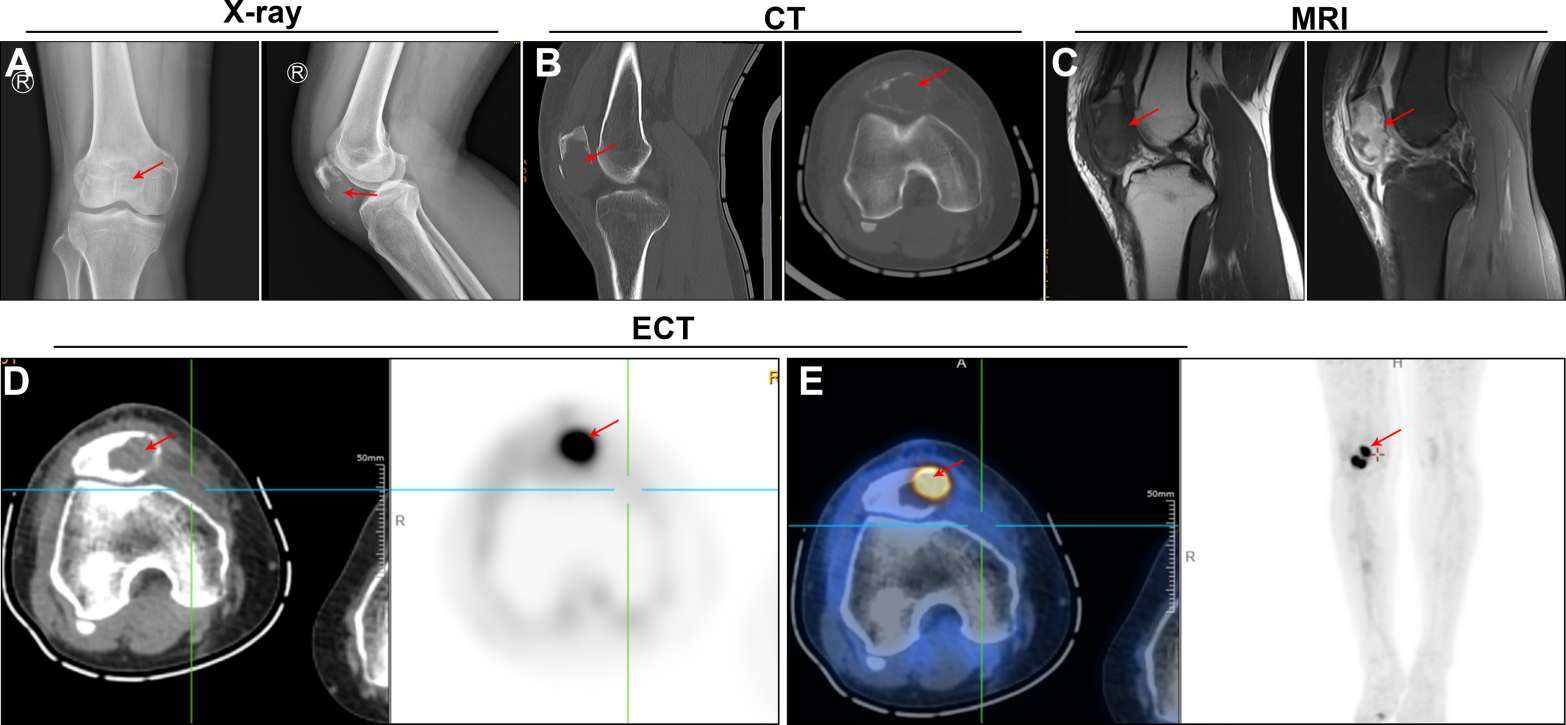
Imaging views of the knee. (A) Anterior and lateral X-ray views showed the osteolytic changes (red arrows) in the patella. (B) Sagittal and transverse computed tomography (CT) images showed the decreased bone density (red arrows) in the patella, and tumor involvement inside the whole patella. (C) Sagittal magnetic resonance imaging (MRI) revealed a low signal in the T1-weighted image and a high signal in fat-suppressed T2-weighted image (red arrows). (D, E) Positron emission tomography-computed tomography (PET/CT) showed extremely intense focal uptake in the right knee joint and no focal uptake in other sites.
